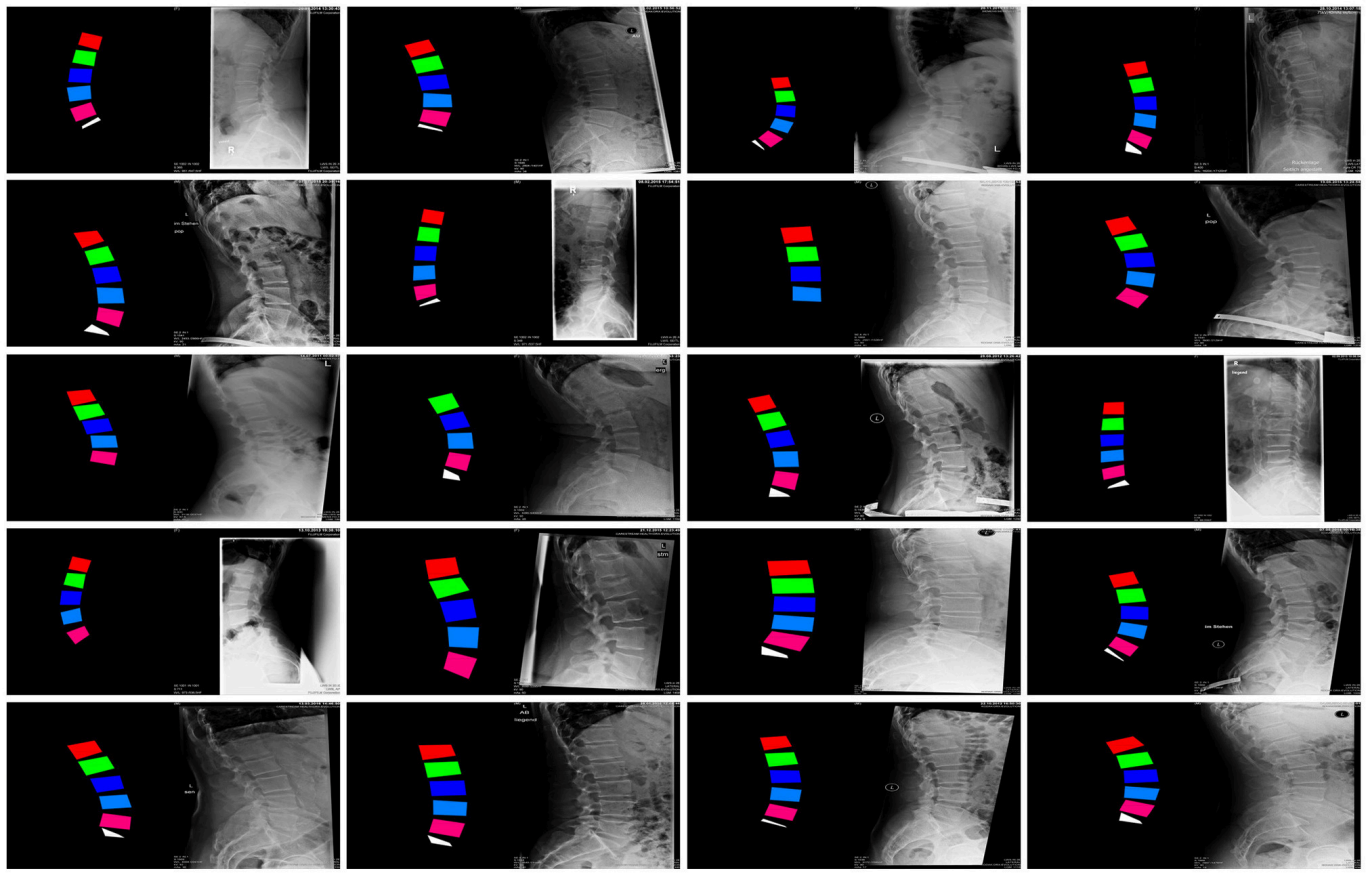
Examples of images in the training dataset used to train the GANs for the generation of synthetic planar X-rays from labels.
